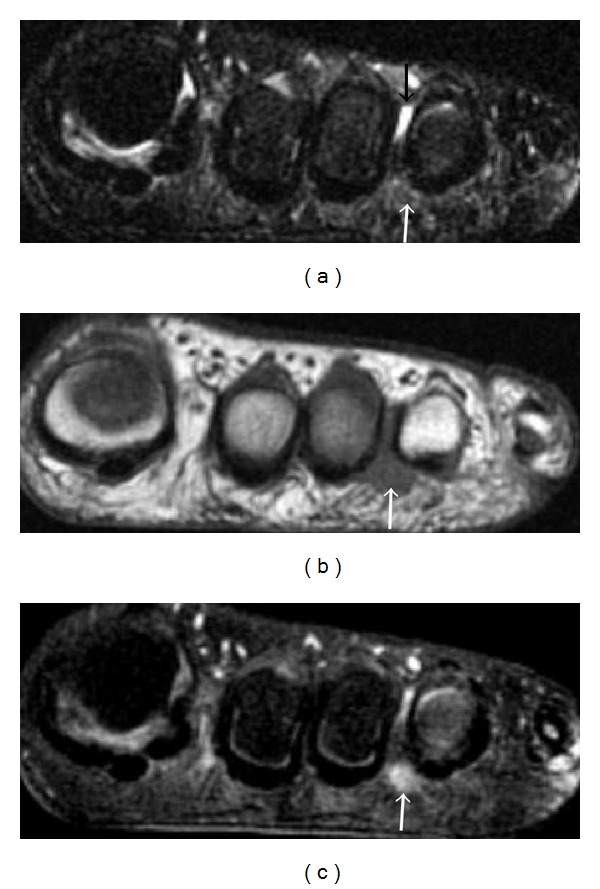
Morton neuroma in a 38-year-old patient. Coronal T2-weighted with fat-saturation (a), T1-weighted (b), and T1-weighted fat-saturated postcontrast (c) images identify an enhancing tear-drop-shaped soft tissue mass (white arrows) with intermediate signal on both T1- and T2-weighted images in the third intermetatarsal space. A small amount of fluid (black arrow) is noted within the intermetatarsal bursa dorsal to the neuroma in (a).
